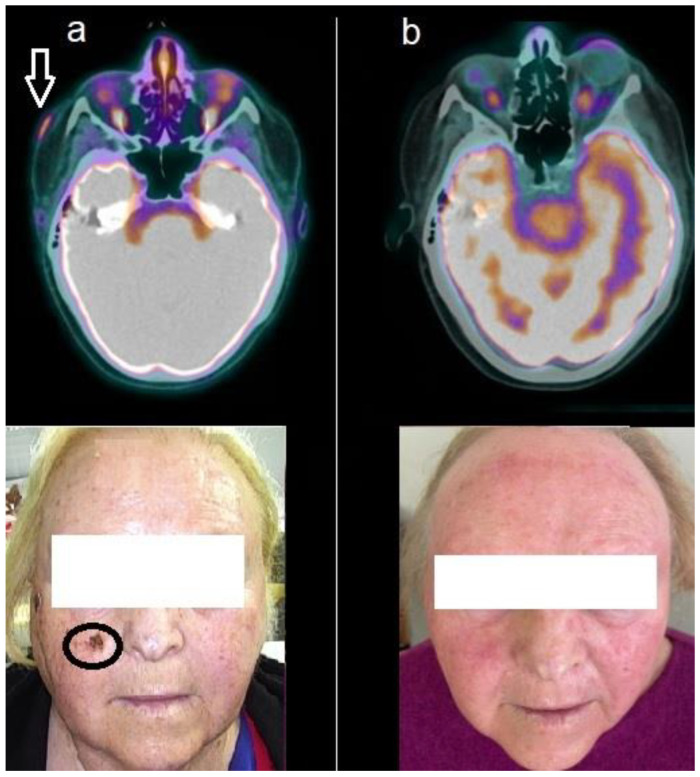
Patient n. 1, a 74-year-old woman, affected by advanced BCC. (a) Baseline ^18^F-FDG PET/CT showed increased tracer incorporation into the lesion of the right zygomatic region, as well evident in the fused axial (upper row, arrow) and at clinical examination (lower row, circle); (b) Follow-up PET/CT acquired after 8 months of HPI therapy, depicted complete metabolic response as evident in the axial images (upper row) and at clinical examination (lower row).
